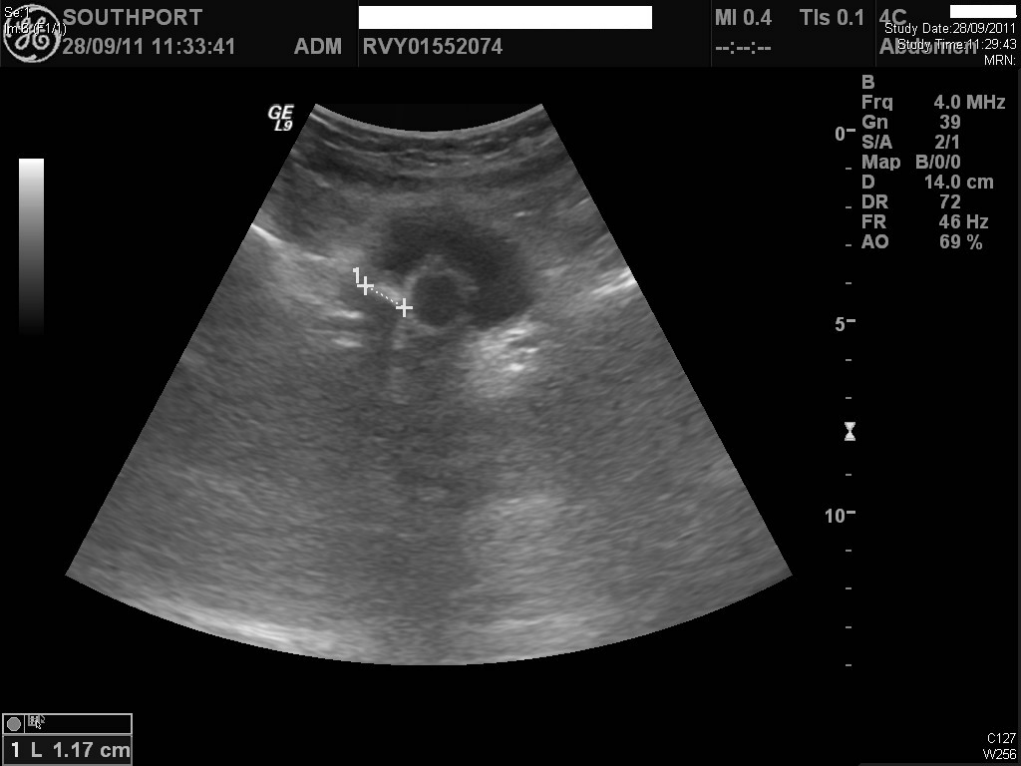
Case number: 3. Ultrasound examination of urinary bladder, performed on 28 September 2011, revealed a 1.2 cm diameter calculus lying to the right side of the balloon of Foley catheter in the bladder. This patient developed autonomic dysreflexia during lithotripsy, which was performed under sedation.
